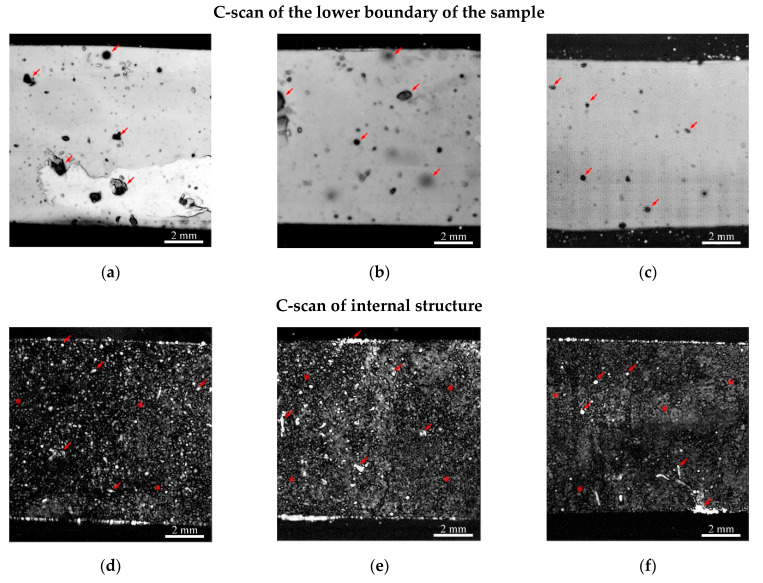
Ultrasonic images of the lower boundary of the initial samples: LDPE/NR 90/10 (a), LDPE/NR 70/30 (b), LDPE/NR 50/50 (c); internal structure of samples: LDPE/NR 90/10 (d), LDPE/NR 70/30 (e), LDPE/NR 50/50 (f). The bright and dark areas marked with arrows represent internal air inclusions, both with and without filler aggregates. The asterisks represent areas of uniform filler distribution, which small particles are represented by gray highlights.
